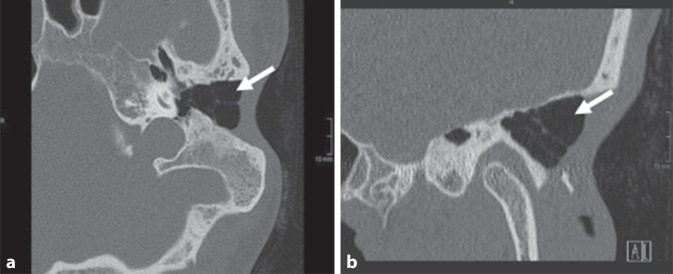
Felsenbein-CT: Die in der kürzlich durchgeführten MRT-Untersuchung als rezidivcholesteatomverdächtige Läsion beschriebene Raumforderung stellt sich sowohl in axialer (a) wie auch in koronaler (b) Ebene als ein luftgefüllter Hohlraum dar (*weißer Pfeil*)
